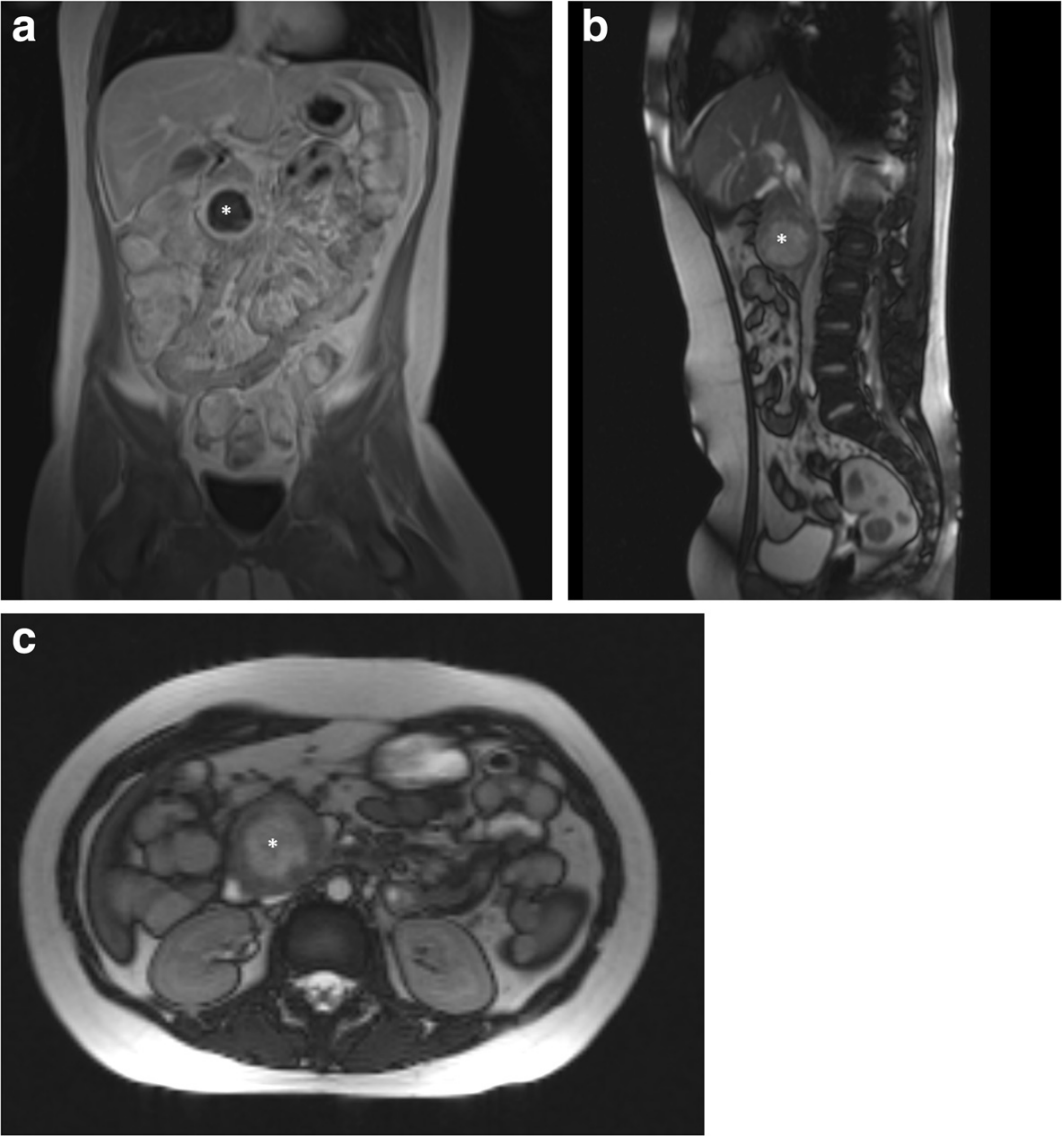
MRI scans in coronary. * SPN. a sagittal b tranversal c reconstruction
